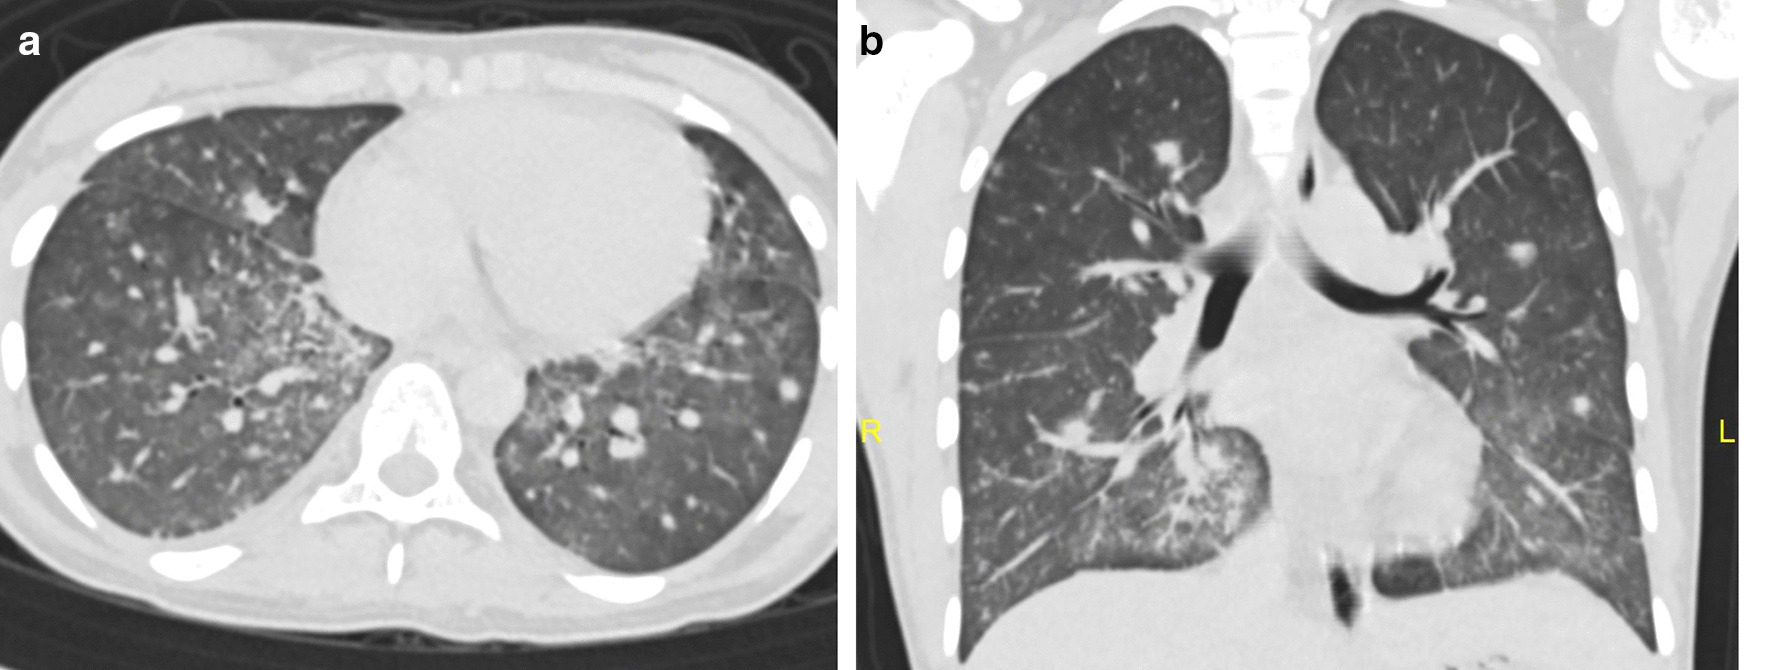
a Axial computed tomography (CT) scan showing bilateral pulmonary parenchymal nodules and ground-glass changes in the middle lobe, lingular segment of left upper lobe and bilateral lower lobes. Upper lobes are less affected. b Coronal CT scan showing bilateral pulmonary parenchymal nodules and ground-glass changes in the middle lobe, lingular segment of left upper lobe and bilateral lower lobes. Upper lobes are less affected
